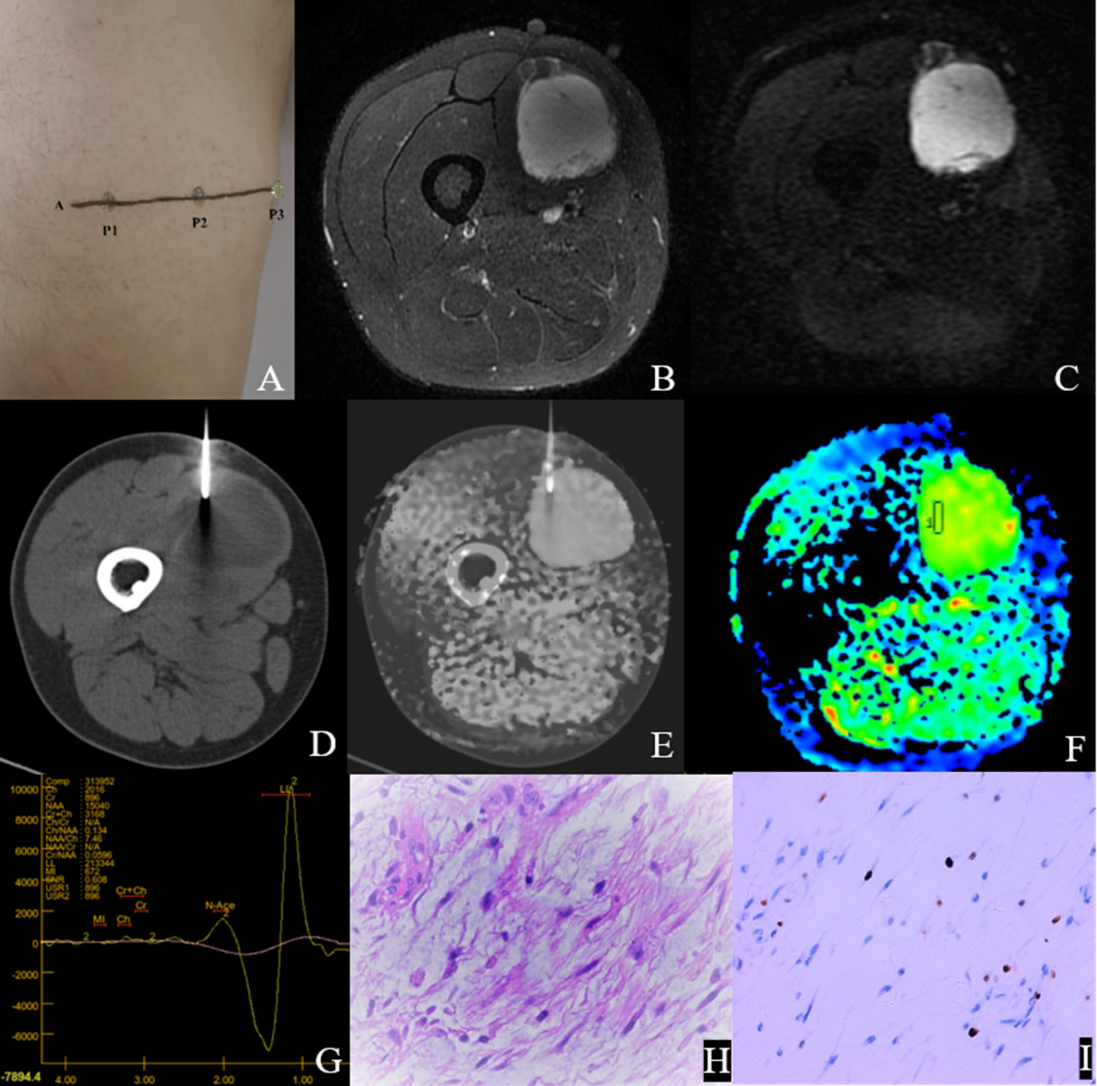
Male,35Y, Myxofibrosarcoma of the right calf, G1. (A) Body surface positioning marks before MRI examination; (B, C) Targeted slice, chosen by Axial T2WI-FS and ADC image; (D) CT guided biopsy at target slice; (E) CT-MRI image fusion at target slice; (F) Draw ROI to obtain DWI quantitative parameters, ADCmin: 3.280×10–^3^ mm^2^/s, ADCmean: 3.370×10–^3^ mm^2^/s; (G)
